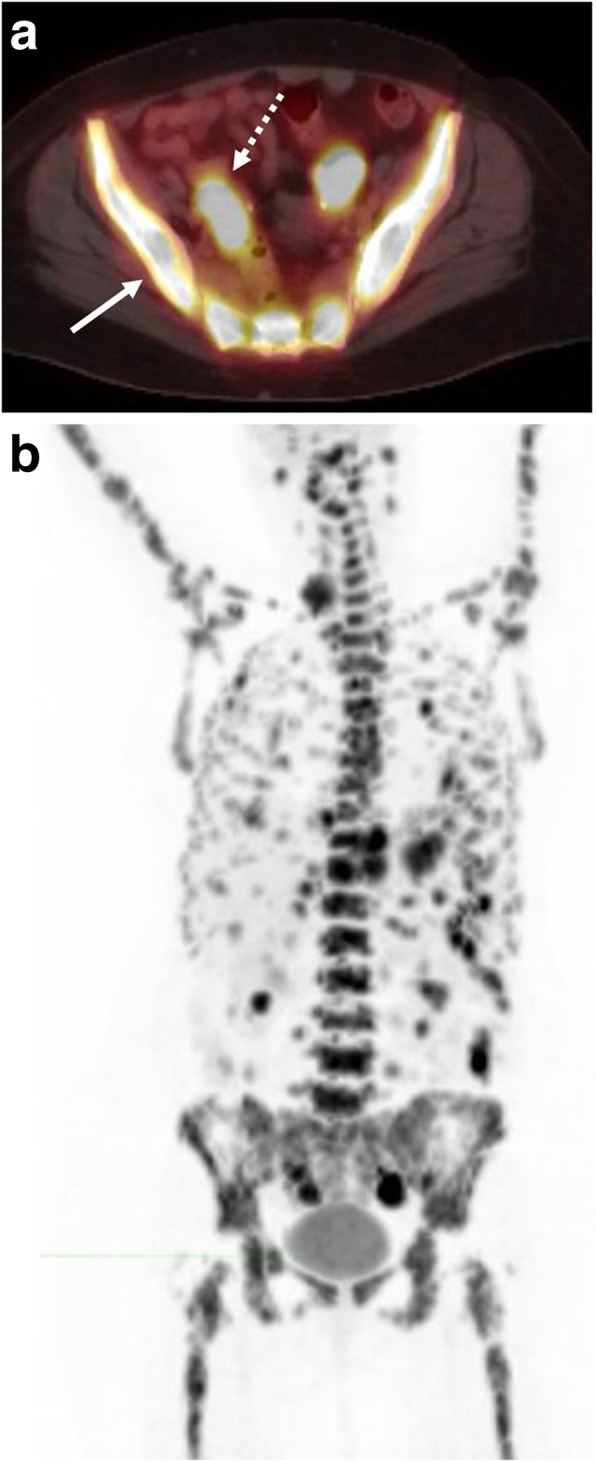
Diffuse marrow involvement. A 74-year-old female with known Burkitt lymphoma. a Fused axial PET-CT demonstrates diffuse hypermetabolic activity within the visualized marrow of the pelvis (solid arrow). Soft tissue activity is also seen within the pelvis (dashed arrow). b Whole body planar PET image demonstrates both diffuse marrow and soft tissue disease within the lower neck, thorax, abdomen, and pelvis
